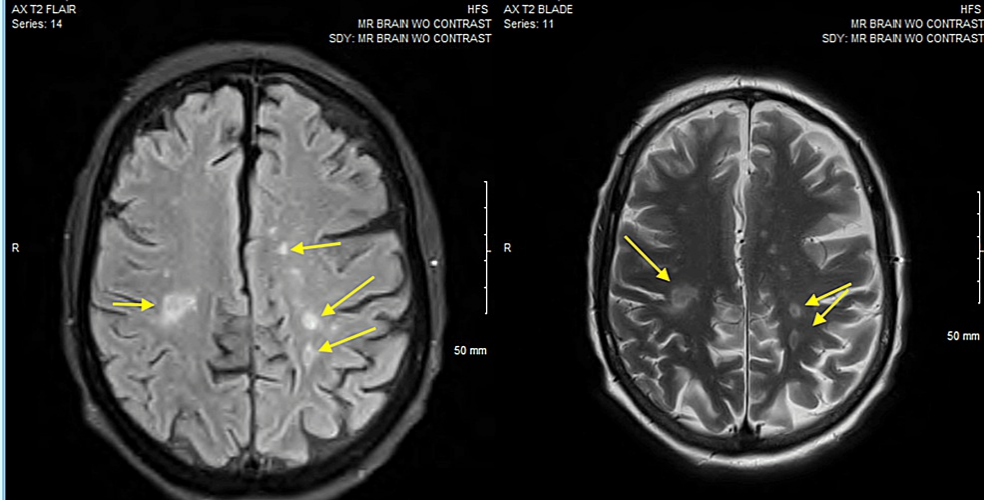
Brain MRI showing multiple infarcts throughout the cerebral hemispheres suggestive of septic emboli
